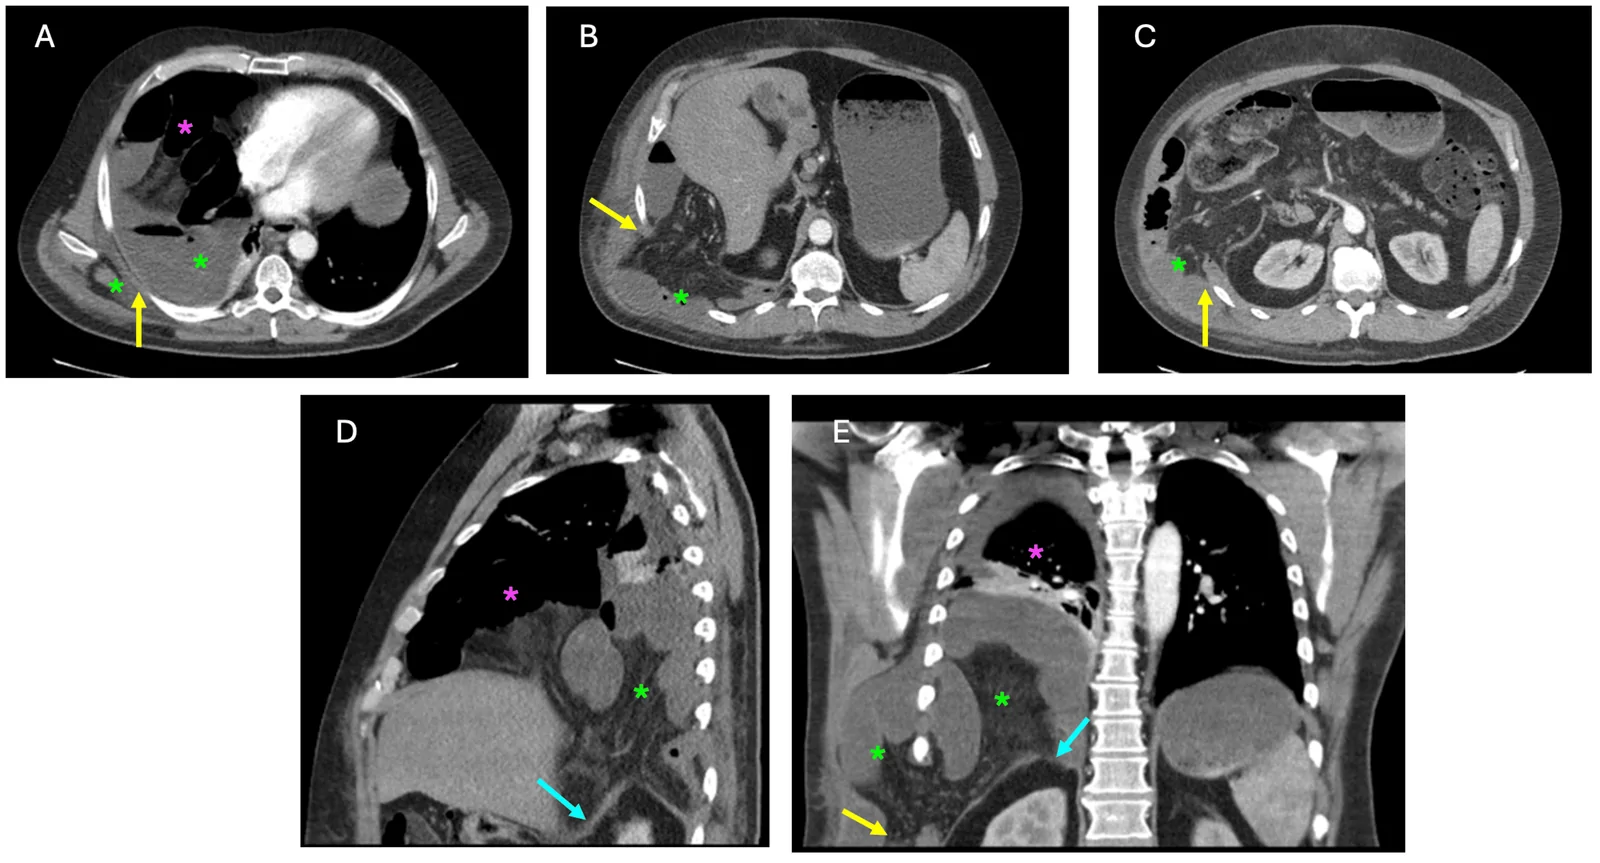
CT findings of the right superior lumbar hernia Axial (A–C), sagittal (D), and coronal (E) contrast-enhanced CT images demonstrate the right posterolateral abdominal wall hernia. Yellow arrows indicate the hernia defect; green asterisks indicate herniated bowel/hernia contents with surrounding fluid; cyan arrows indicate the intact diaphragm; and magenta asterisks indicate the adjacent lung. Multiplanar images (D–E) demonstrate the relationship of the hernia to the intact diaphragm.
